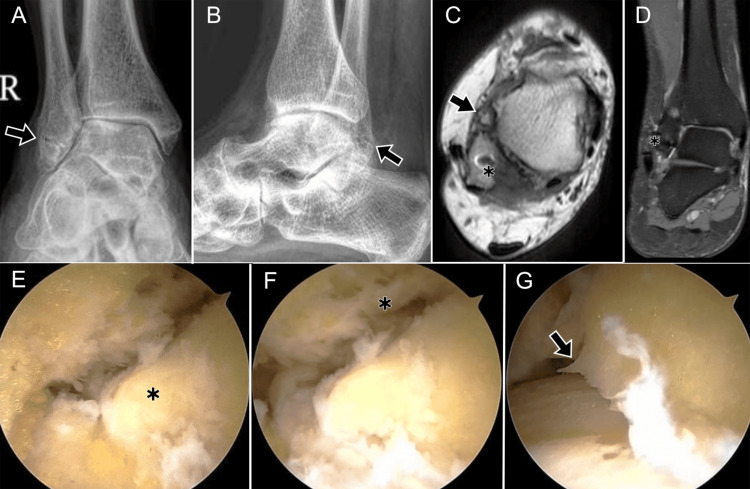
Chronic instability and tibio-talar osteoarthritis. A 66-year-old male: (A, B) anteroposterior and lateral weight-bearing radiographs show sclerosis, surface irregularity, and marginal osteophytes indicating osteoarthritis (arrows); (C, D) axial DP MRI (double-echo proton) and coronal STIR MRI reveal distal fibula surgical changes (asterisk), osteophytes, an ossicle in the ATFL recess, and a full-thickness ATFL tear (arrow); (E-G) arthroscopy shows synovial thickening (asterisk), tibiofibular instability (variable amplitude), irregular cartilage, and marginal osteophytes (arrow). STIR: short tau inversion recovery; ATFL: anterior talofibular ligament Images belong to the authors; patient consent was obtained.
